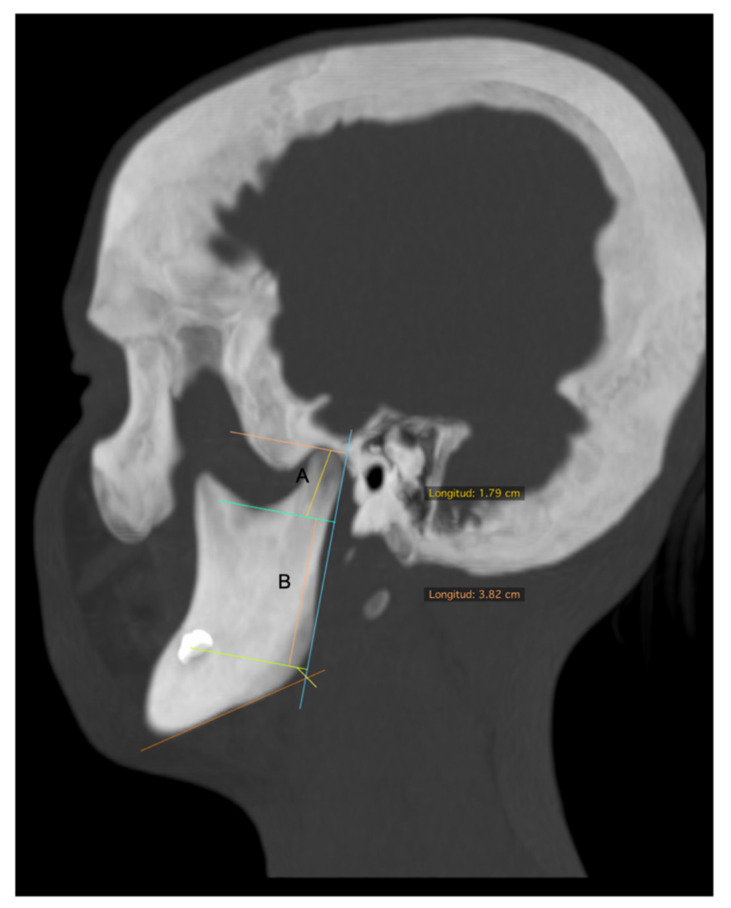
Bone tissue CT, sagittal view. (A) Condylar length. (B) Mandibular ramus length.
